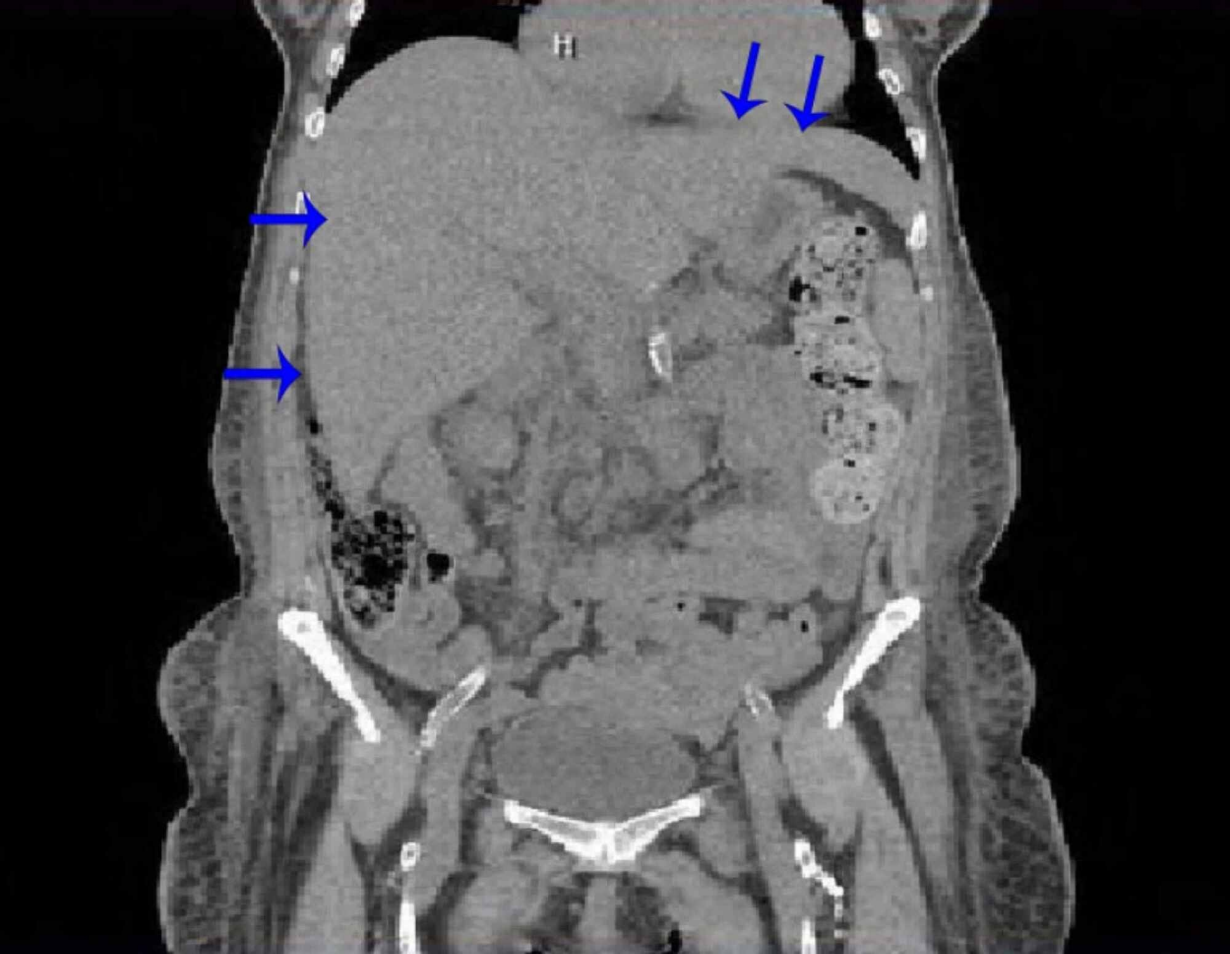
CT of the abdomen showing hepatomegaly
